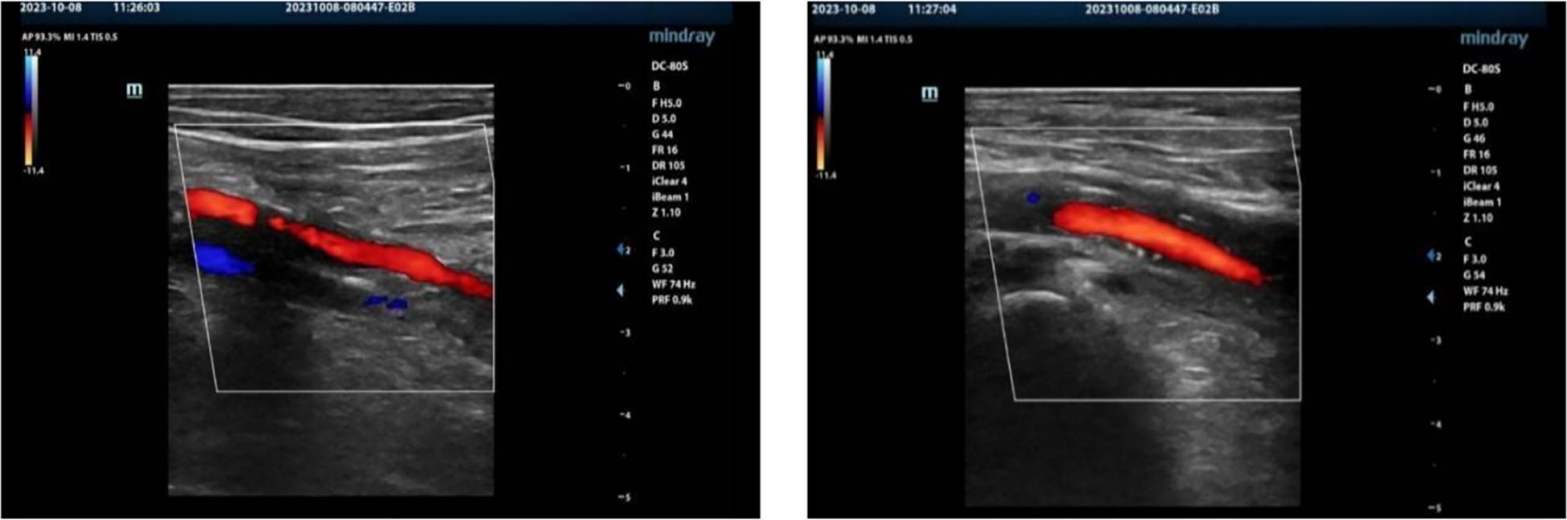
Ultrasound indicating stenosis of the lower segment of the left superficial femoral artery, deep femoral artery, popliteal artery, and posterior tibial artery. Closure of the left anterior tibial artery and the common peroneal artery. Normal blood flow in the veins of the left lower extremity.
